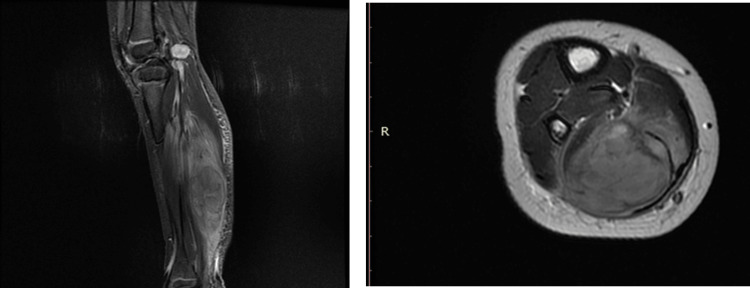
MRI with contrast of the diagnosis (coronal, axial)
